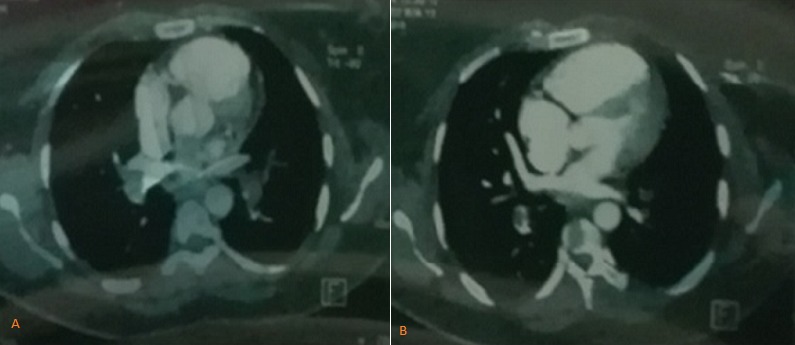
Chest CT scan showing proximal pulmonary embolism (A) and dilated right ventricle (B)
